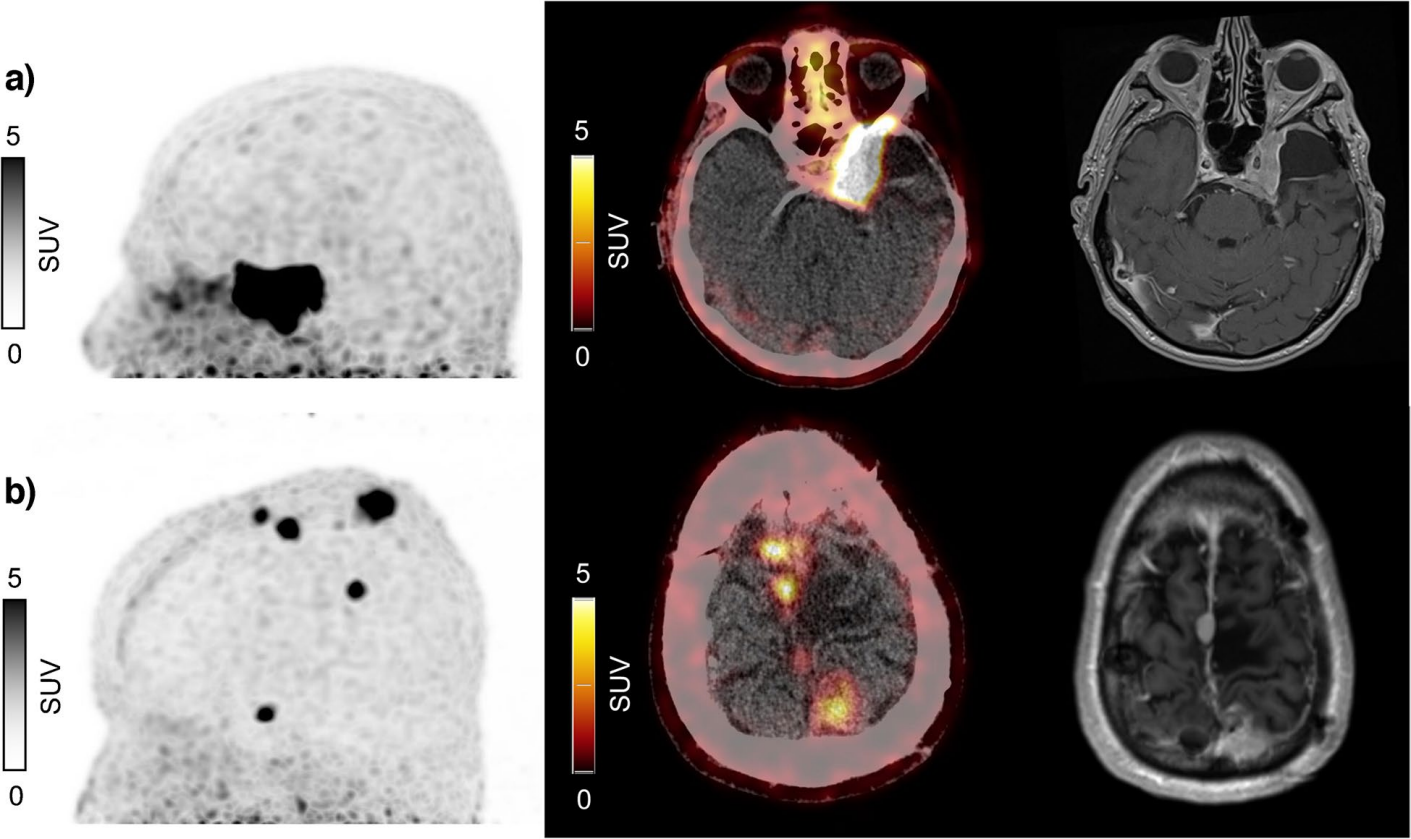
Left: Maximum intensity projection (MIP) representation of DOTATOC PET scan in lateral view. Middle: transversal slice of PET Scan after fusion with low-dose CT scan. Right: Transversal slice of pretherapeutic MRI (T1 post KM) (a) Example of a patient with a single lesion and (b) a patient with multiple lesions
